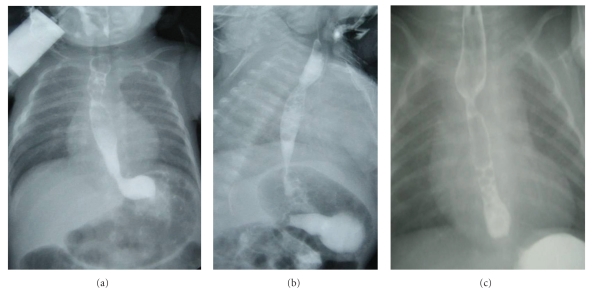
(a) Normal esophageal passage X-ray view of the case with no anastomotic stricture. (b) Nonsymptomatic moderate narrowing and (c) serious anastomotic stricture that caused expansion of the proximal esophagus (another case of our patients).
